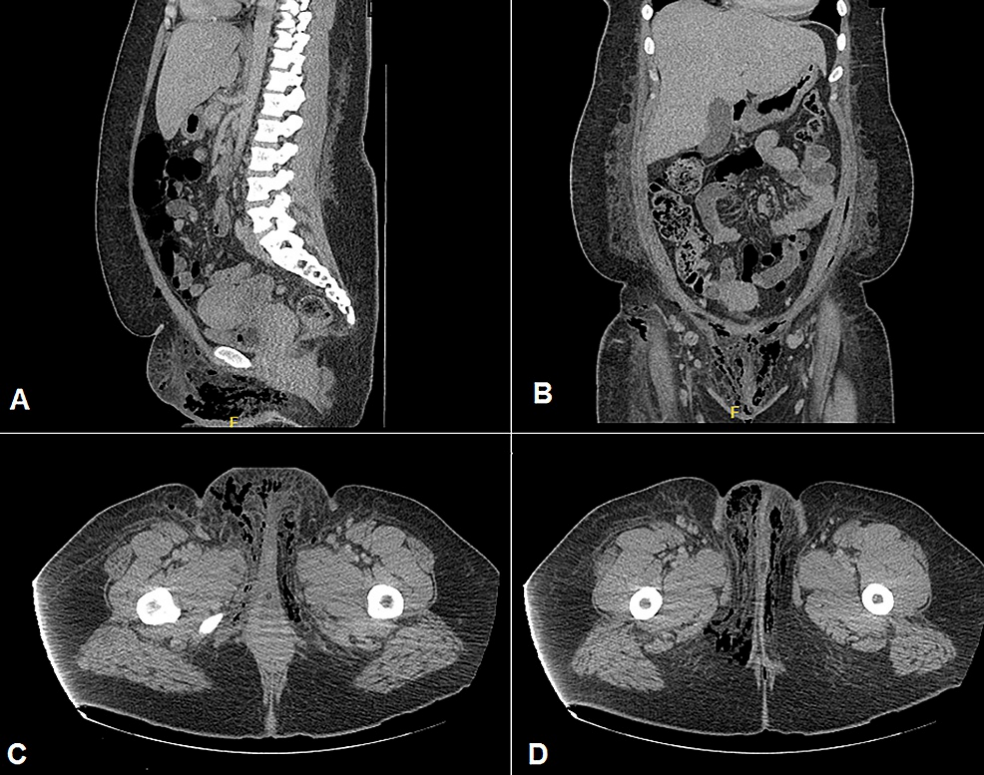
CT of abdomen and pelvis with contrast demonstrating subcutaneous emphysema in the lower abdominal and pubic region in (A) sagittal and (B) coronal sections; in bilateral perineum, labia and groin region in axial sections (C, D).
